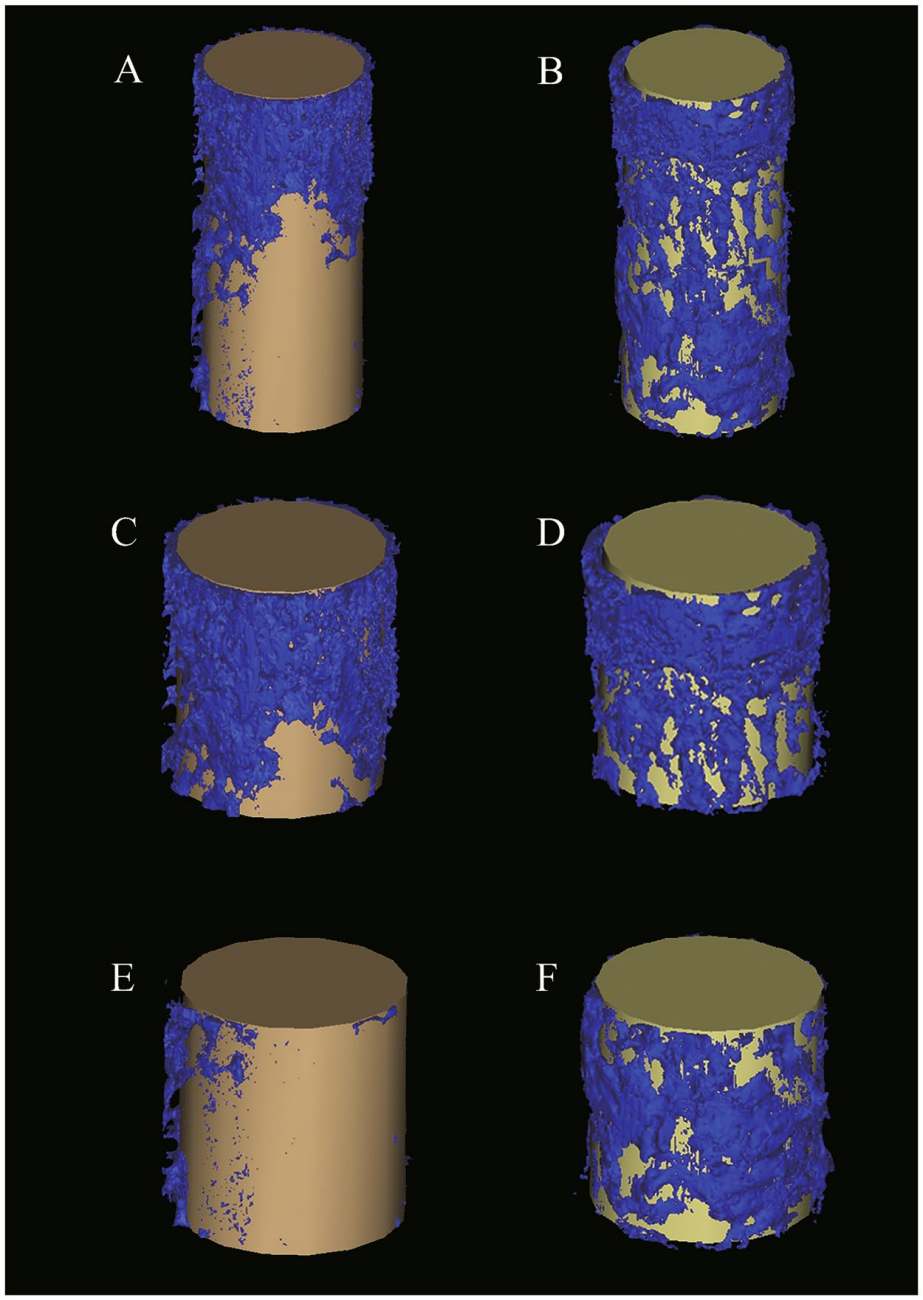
Representative 3D representations of micro-computed tomography images of implant and bone volume around implants at 4 weeks. Micro-computed tomography images of (A) total titanium implants, (B) total PEO-coated implants, (C) proximal titanium implants, (D) proximal PEO-coated implants, (E) distal titanium implants, and (F) distal PEO-coated implants. PEO, plasma electrolytic oxidation.
